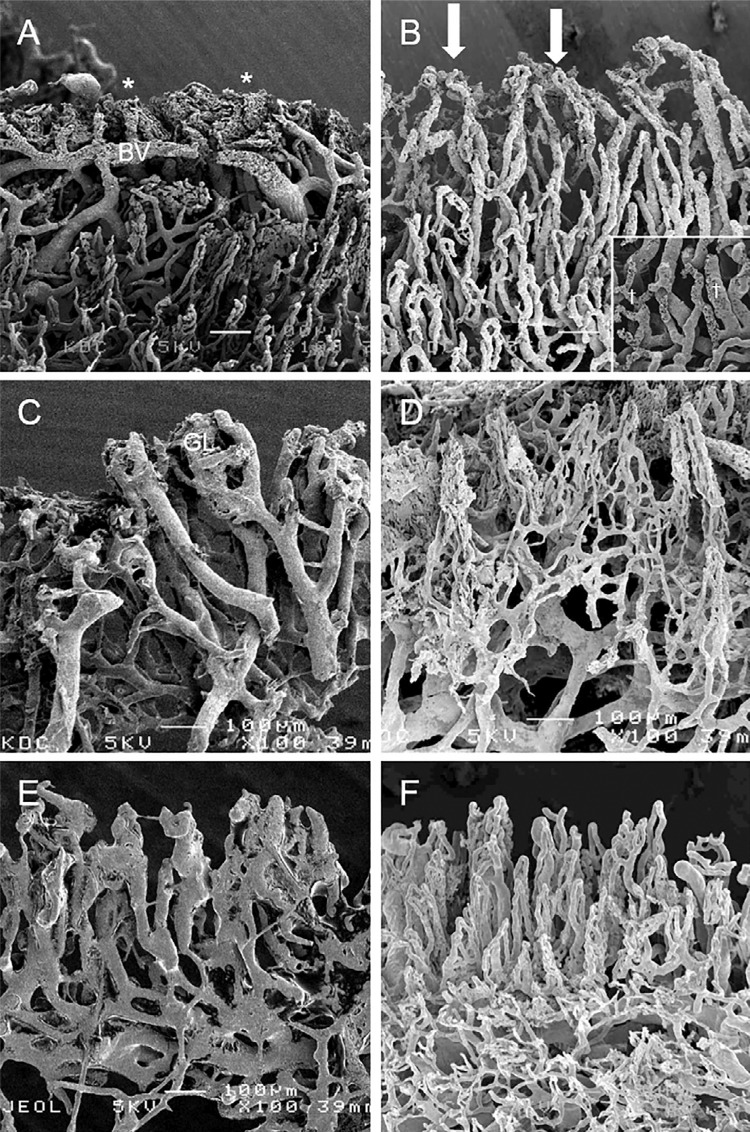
Vascular loops of the gingival margin in the turbine and ultrasonic group. A: On Day 0, in the turbine group, the injected resin flowed from the vessels (*), and main vessels (BV) in the deeper areas are on the gingival surface. B: On Day 0, in the ultrasonic group, the vascular networks are quite similar to those of the control group. U-turn loops were visible in the marginal gingiva (arrows). Round concavities (†) on the surface of blood vessels indicate the presence of red blood cells (square frame). C: On Day 7, in the turbine group, the vessels of the marginal gingiva were thicker in diameter (30–90 µm; GL) and glomerular loops were formed. D: On Day 7, in the ultrasonic group, thin U-turn loops exist in the marginal gingiva. These loops indicate the blood vessels regeneration . E: On Day 30, in the turbine group, blood vessels consisting of flat and thick loops are in the marginal gingiva. F: 30 days after tooth preparation in the ultrasonic group, vascular networks were arranged in a nearly normal manner
